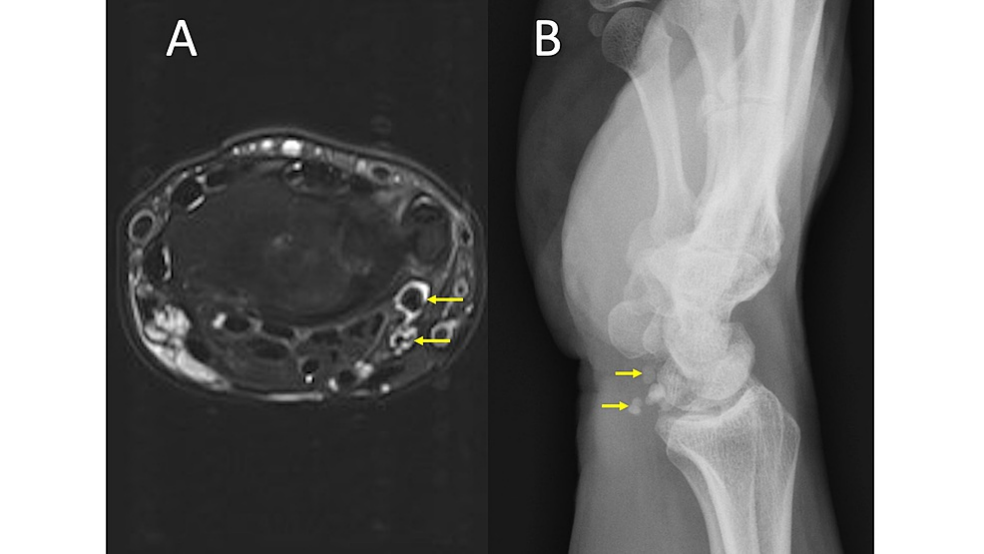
Synovial Osteochondromatosis MRI axial T2 fat saturation (FS) (12A) shows multiple small, round, hypointense, similar-sized foci along the volar wrist on both the radial and ulnar sides surrounded by fluid (yellow arrows). Lateral radiograph of the wrist (12B) demonstrates multiple mineralized bodies along the volar aspect of the wrist (yellow arrows).
